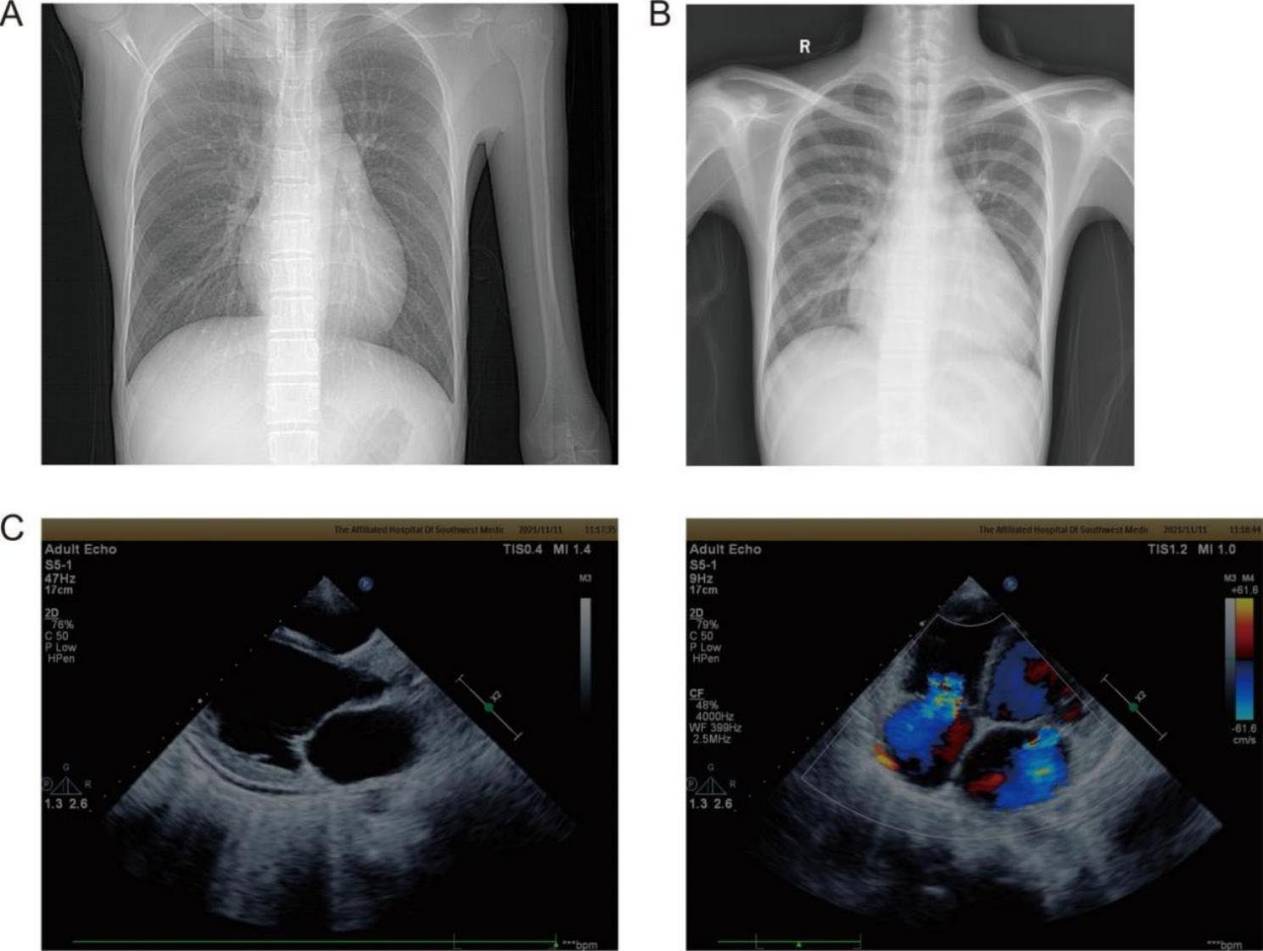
Chest X-ray and transthoracic echocardiography of the patient. (A) The chest X-ray of the patient showed a normal heart size at first admission. (B) The chest X-ray demonstrated an enlarged cardiac silhouette after 17 months. (C) Echocardiography revealed biventricular enlargement and moderate regurgitation of mitral and tricuspid valves after 17 months
